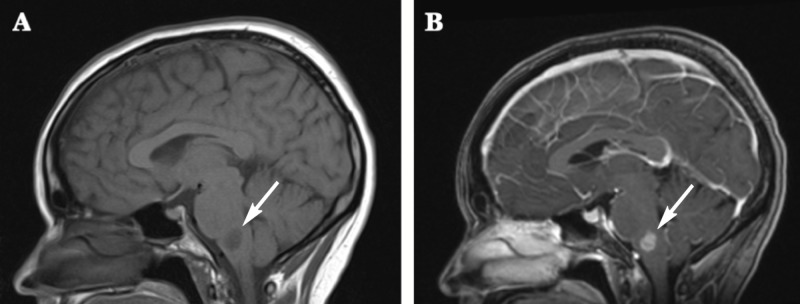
Magnetic resonance imaging (MRI) prior to biopsy. The lesion within the medulla (arrows) demonstrated restricted diffusion and smooth, moderately thick peripheral enhancement with central necrosis (A: T1-weighted image, B: T2-weighted image).
